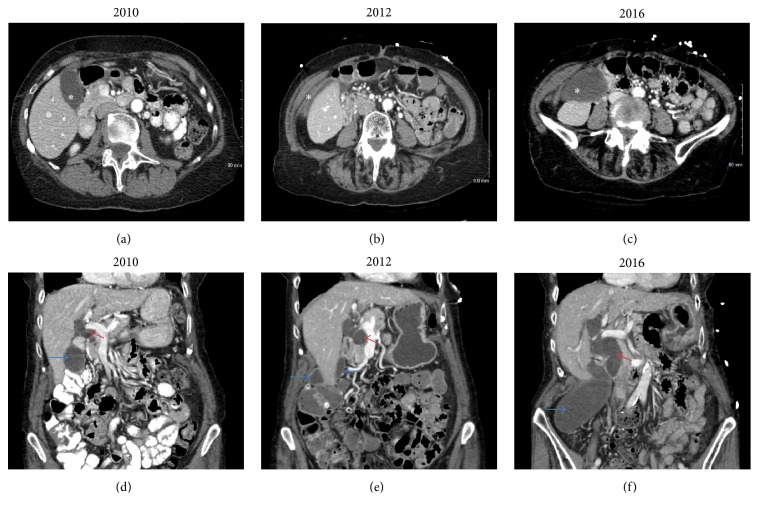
(a), (b), and (c) show computed tomography axial images of the abdomen with the gallbladder marked by (*∗*). Notice the different anatomical positions of the gallbladder over a six-year period. (d), (e), and (f) show computed tomography coronal images of the abdomen showing changes in the anatomical position of the gallbladder (blue arrow); the choledochal cyst (red arrow) is shown to be increasing in size over the same period.
